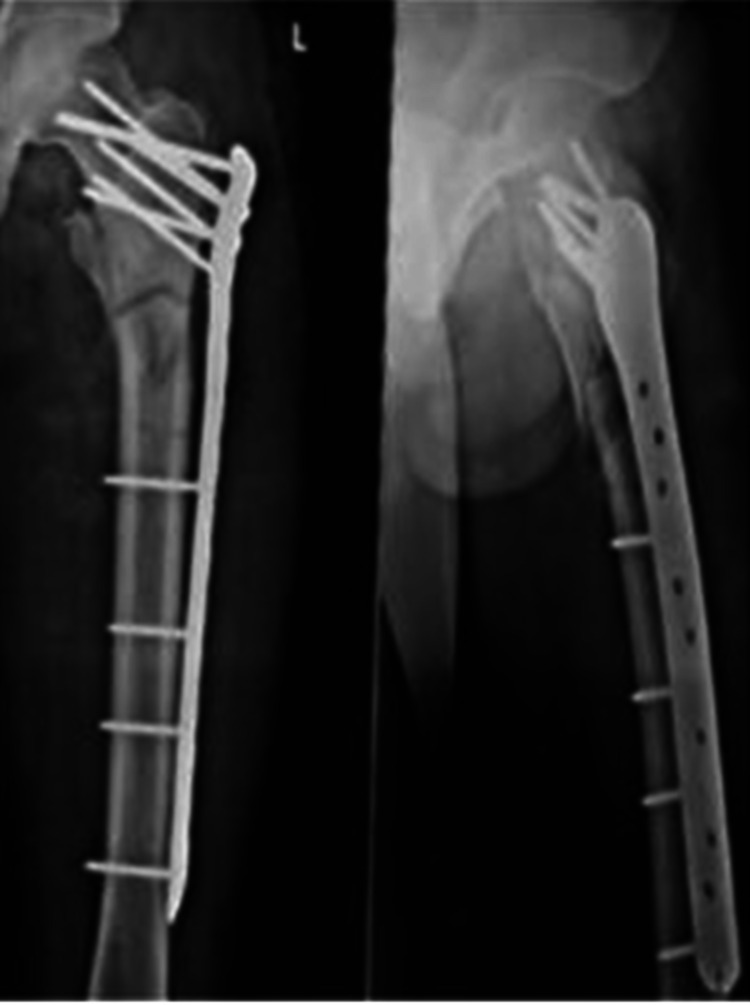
Antero-posterior and lateral radiographs at six weeks of follow-up
